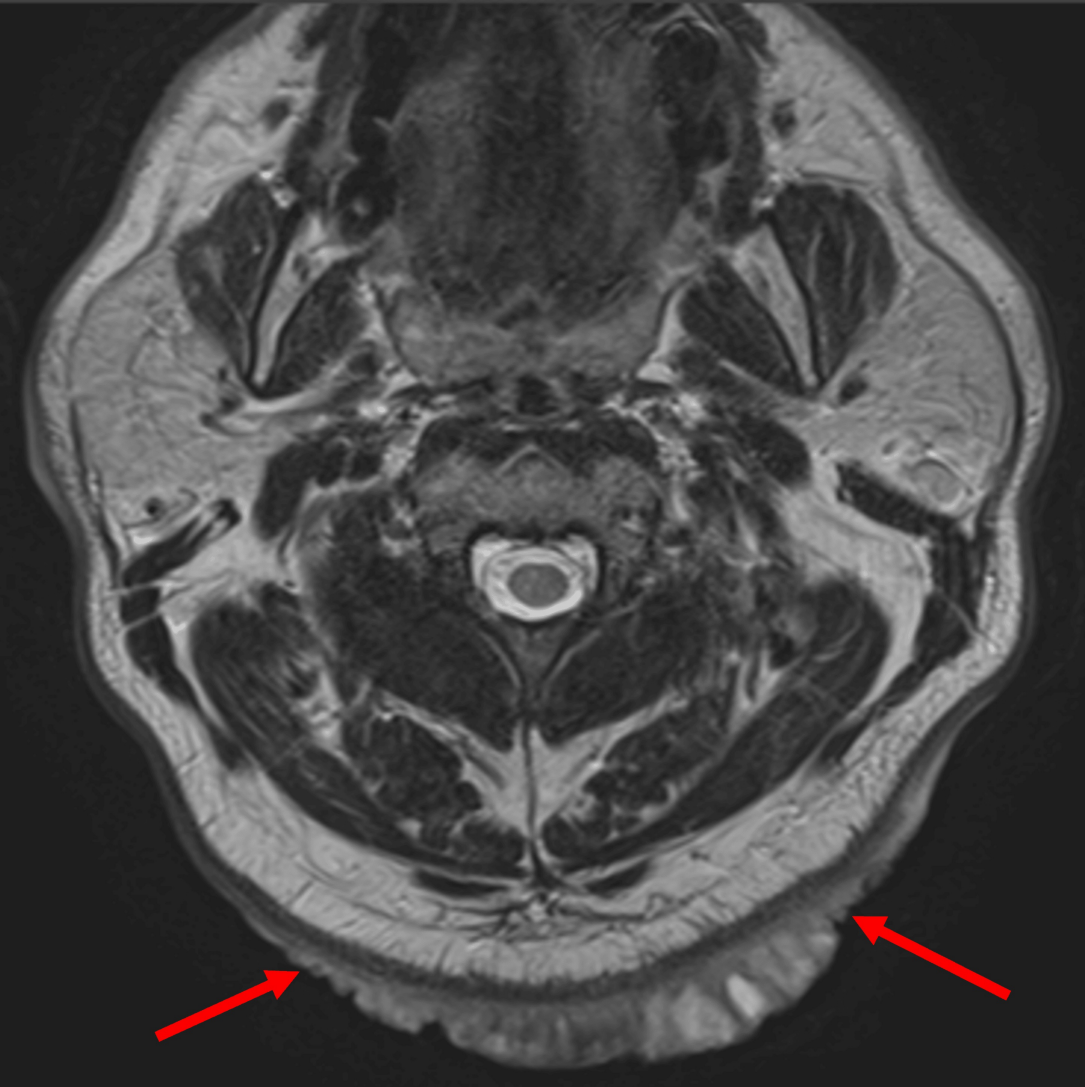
Preoperative MRI T2-weighted magnetic resonance imaging (MRI) showing a high-signal area extensively spreading within the subcutaneous tissue from the occipital region of the scalp to the posterior neck. Infiltration into the subcutaneous fat layer was not observed Red arrow: tumor
